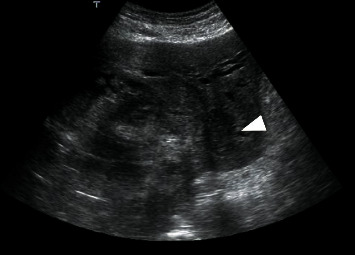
Abdominal ultrasonography showing a 15-mm hypoechoic mass in liver segment S2 adjacent to the bleeding (arrowhead). A percutaneous biopsy was performed.
